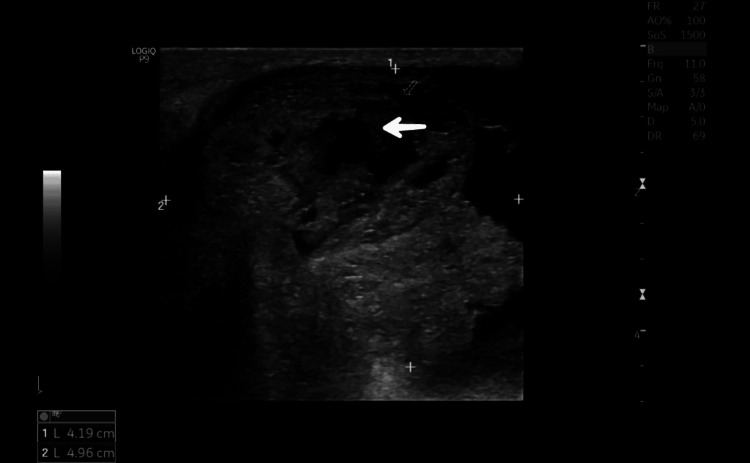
Breast ultrasound image of case 1 Ultrasound of the breast shows a large cystic lesion with solid components with free-floating internal echoes and internal vascularity (arrow) (BI-RADS IV C/V). BI-RADS: Breast Imaging Reporting and Data System
